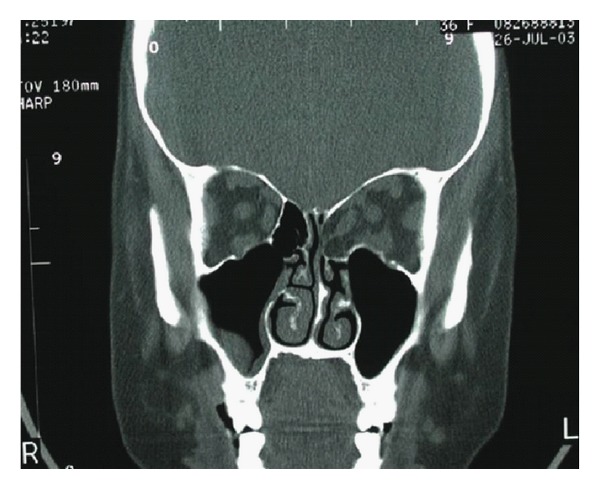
Coronal CT scan shows fat herniation into ethmoid sinus after transcaruncular medial wall orbital decompression.
